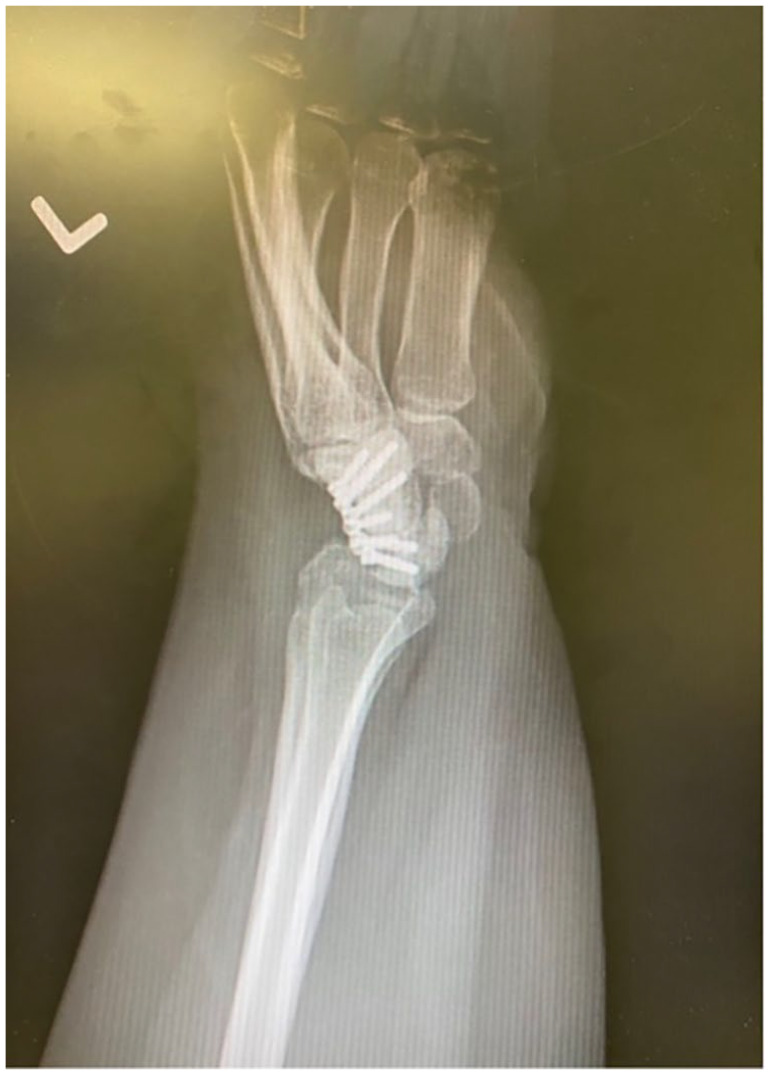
Lateral radiograph of a 4-corner fusion using a circular plate for fixation of the capitate, hamate, lunate, and triquetrum. The scaphoid has been excised.
